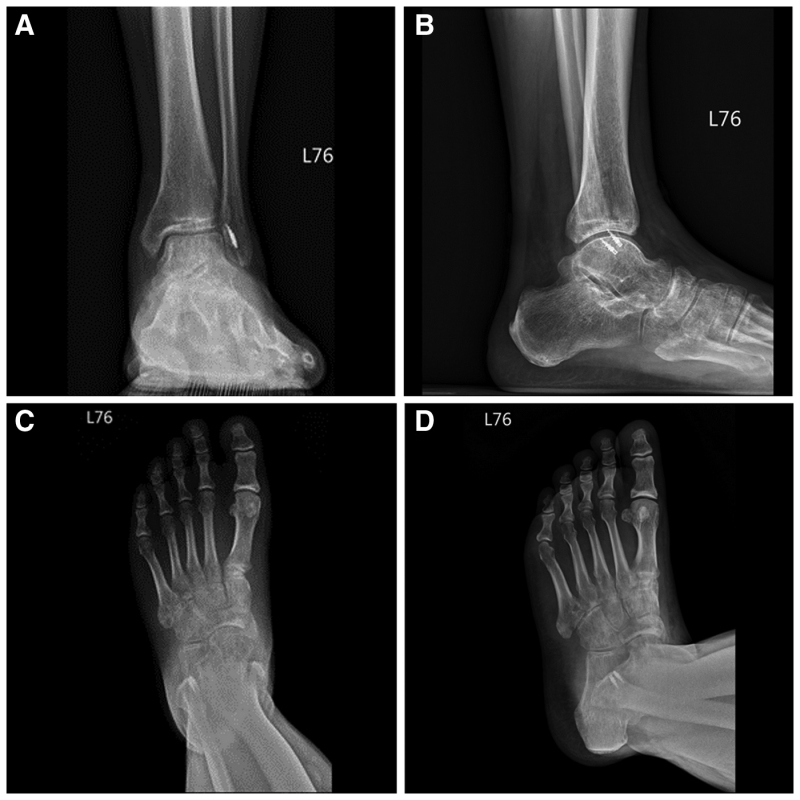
Postoperative 6-mo plain radiographs of the left ankle and foot. (A) Anteroposterior view and (B) lateral view of the left ankle, and (C) anteroposterior view and (D) lateral view of the left foot, demonstrating stable graft positioning and integration.
